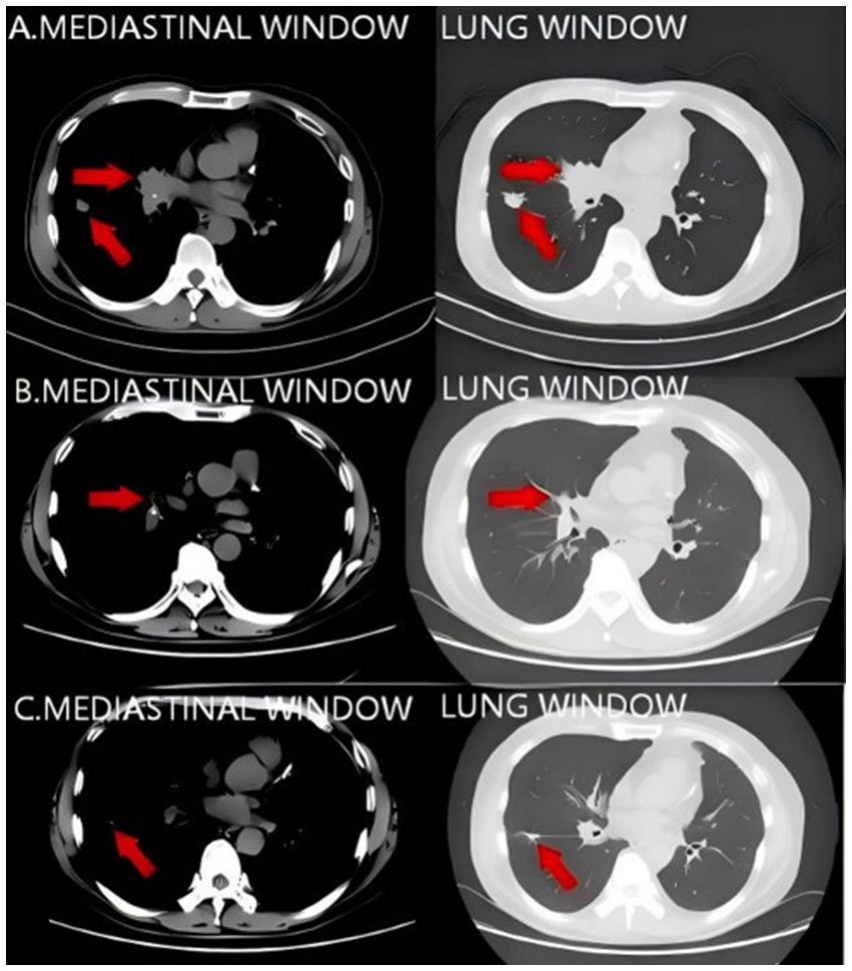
(A) Pulmonary CT findings on December 30, 2020. Slight enlargement of the right pulmonary hilum with consolidation of the right middle lobe, obstruction of the right middle lobe bronchial orifice, and a nodule in the right lower lobe. (B,C) Pulmonary CT findings on May 21, 2021. The pulmonary mass is reduced in size compared with the previous examination. Red arrows indicate the location of the tumor.
